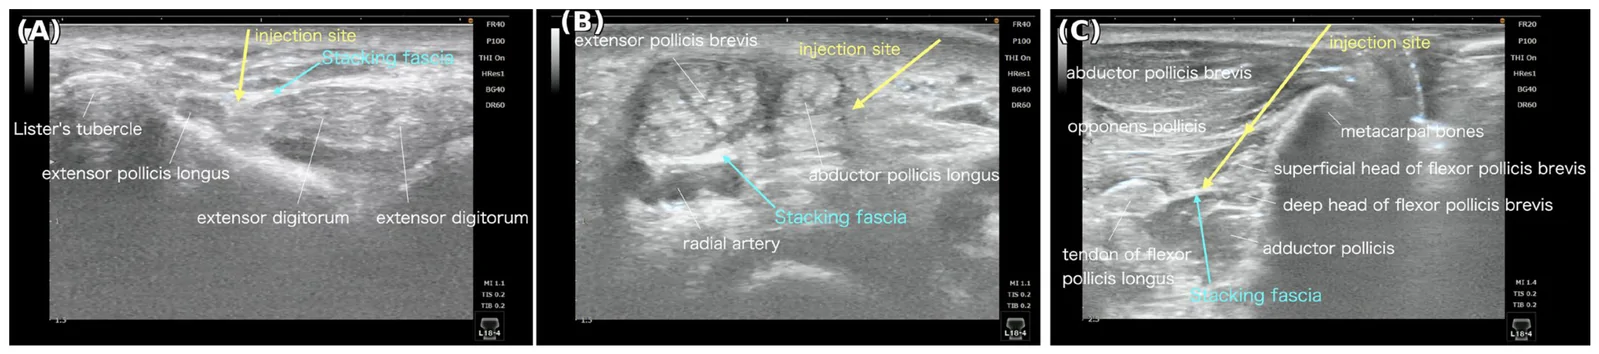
Representative ultrasound images of “stacking fascia” in the thumb–wrist region. Point numbers correspond to the 15-point treatment protocol described in Section 3. (A) Dorsal aspect in the third extensor compartment (Point 2): extensor pollicis longus (EPL) crossing the extensor digitorum tendons at the level of Lister’s tubercle. (B) Vascular crossing in the first extensor compartment (Point 5): radial artery passing adjacent to abductor pollicis longus (APL) and extensor pollicis brevis (EPB). (C) Palmar deep aspect in the thenar region (Point 10): layered thenar muscles (abductor pollicis brevis, opponens pollicis, superficial and deep heads of flexor pollicis brevis, and adductor pollicis) around the metacarpal bones, with the flexor pollicis longus (FPL) tendon visible. Cyan arrows indicate hyperechoic strip-shaped “stacking fascia”; yellow arrows indicate the injection site for ultrasound-guided fascia hydrorelease (US-FHR).
